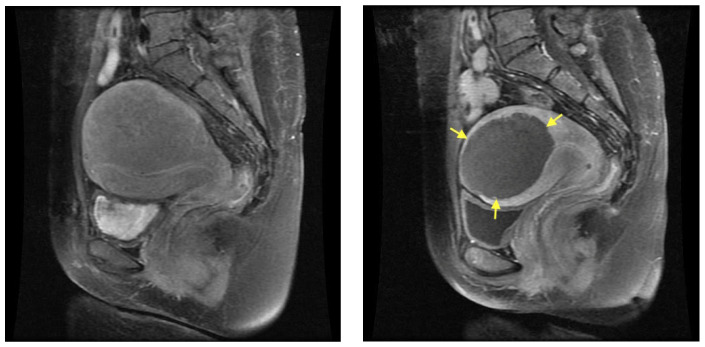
Magnetic resonance (MR) images of adenomyosis from a 47-year-old woman: (Left) pre-HIFU MR image showing extensive adenomyosis involving mostly the posterior fundal uterine wall; (Right) post-HIFU MR image, 6 months after treatment, showing a hypoperfused well-defined area (arrows) as the result of HIFU.
